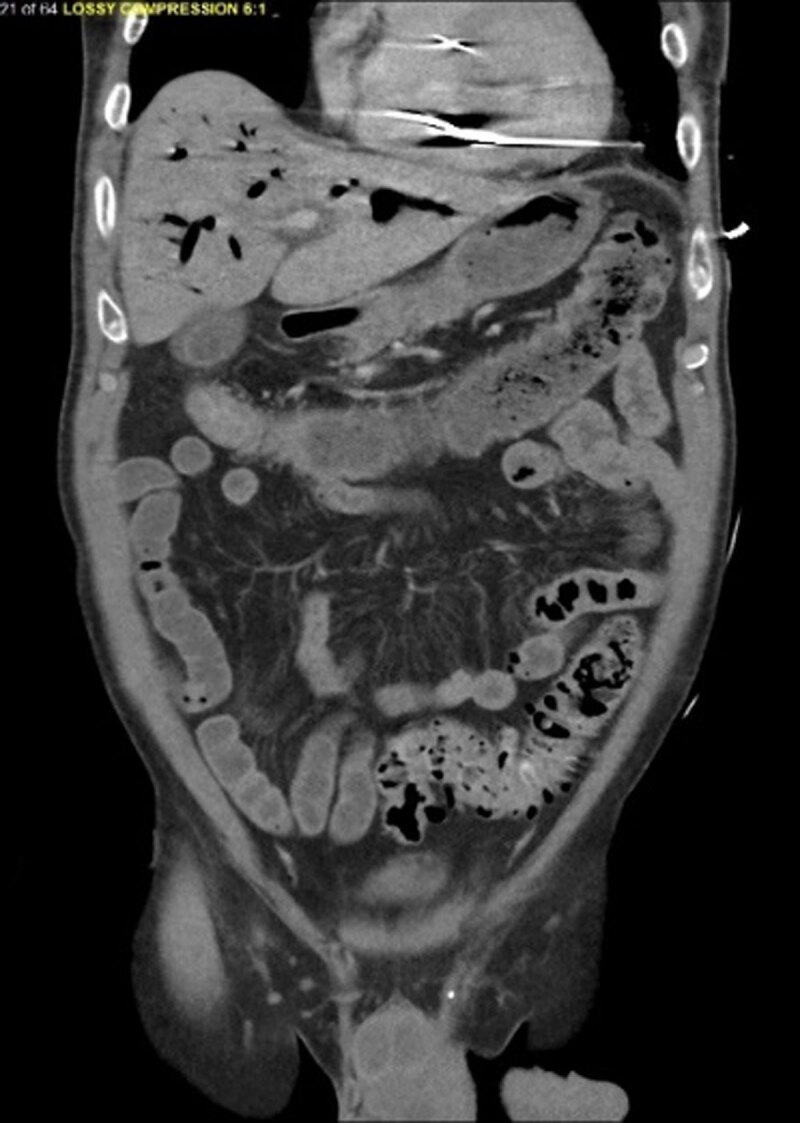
Computed tomography of the abdomen showing a venous air embolism in right ventricle and portal system.
